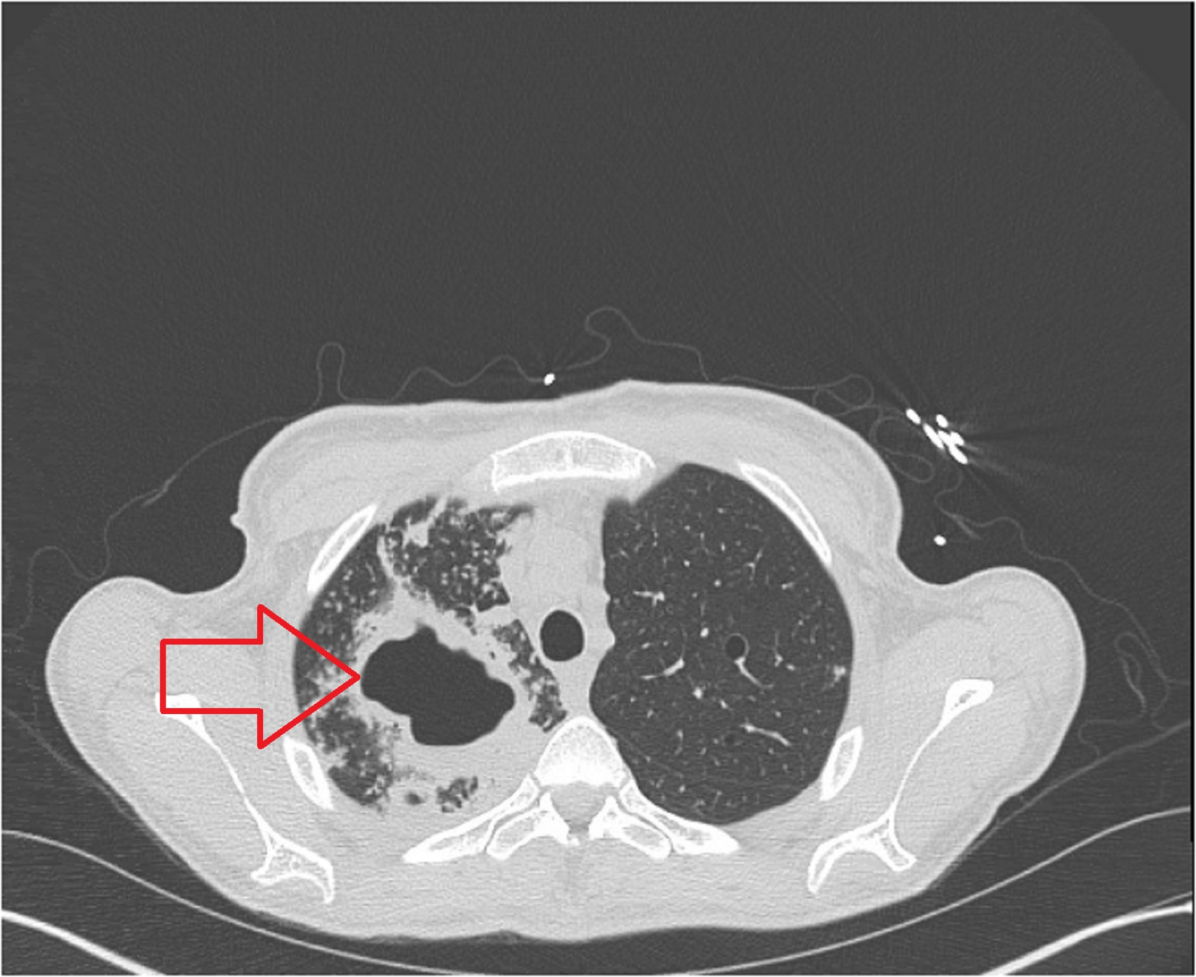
Computed tomography of the patient with tuberculosis. Large cavitation with surrounding area of consolidation.
